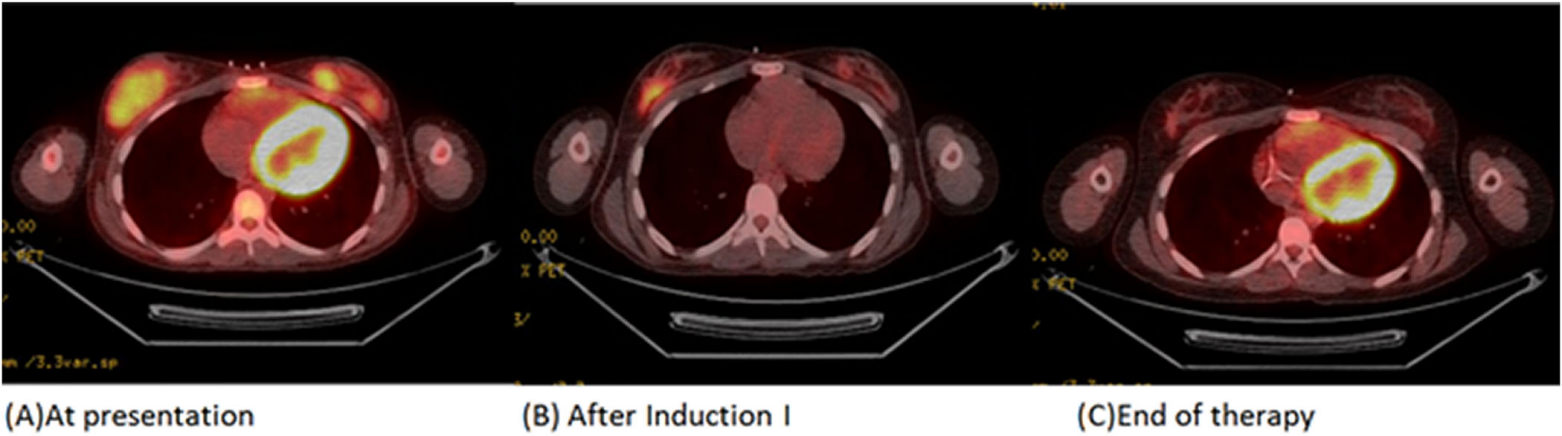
(A) Several nodular masses demonstrated in both breasts with fluorodeoxyglucose (FDG) uptake (standardized uptake value [SUV] max: 2.9 on right breast and SUV max: 2.7 on left breast), thickening over the left chest wall between the posterior medial fifth and sixth ribs with focal increased FDG uptake, and intense FDG uptake in the upper sacrum and sub‐centimeter subcarinal lymph node with mild FDG uptake. (B) Improved appearance of the breast masses with decreased FDG uptake overall (SUV max: 2.1 on right breast and SUV max: 1.9 on left breast), focal area of soft tissue thickening in the left chest wall between the medial fifth and sixth ribs was no longer evident and no longer abnormal tracer uptake in that region, and improved subcarinal adenopathy. (C) No area of abnormal tracer uptake is seen on the left breast but continued appearance of mild increased FDG tracer uptake in the lateral aspect of the right breast (SUV max: 1.8) with no evidence for FDG avid adenopathy.
